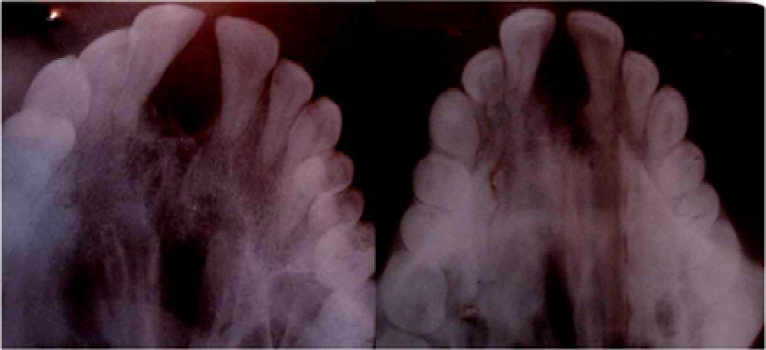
Occlusal radiograph revealed the unilocular appearance of the lesion
